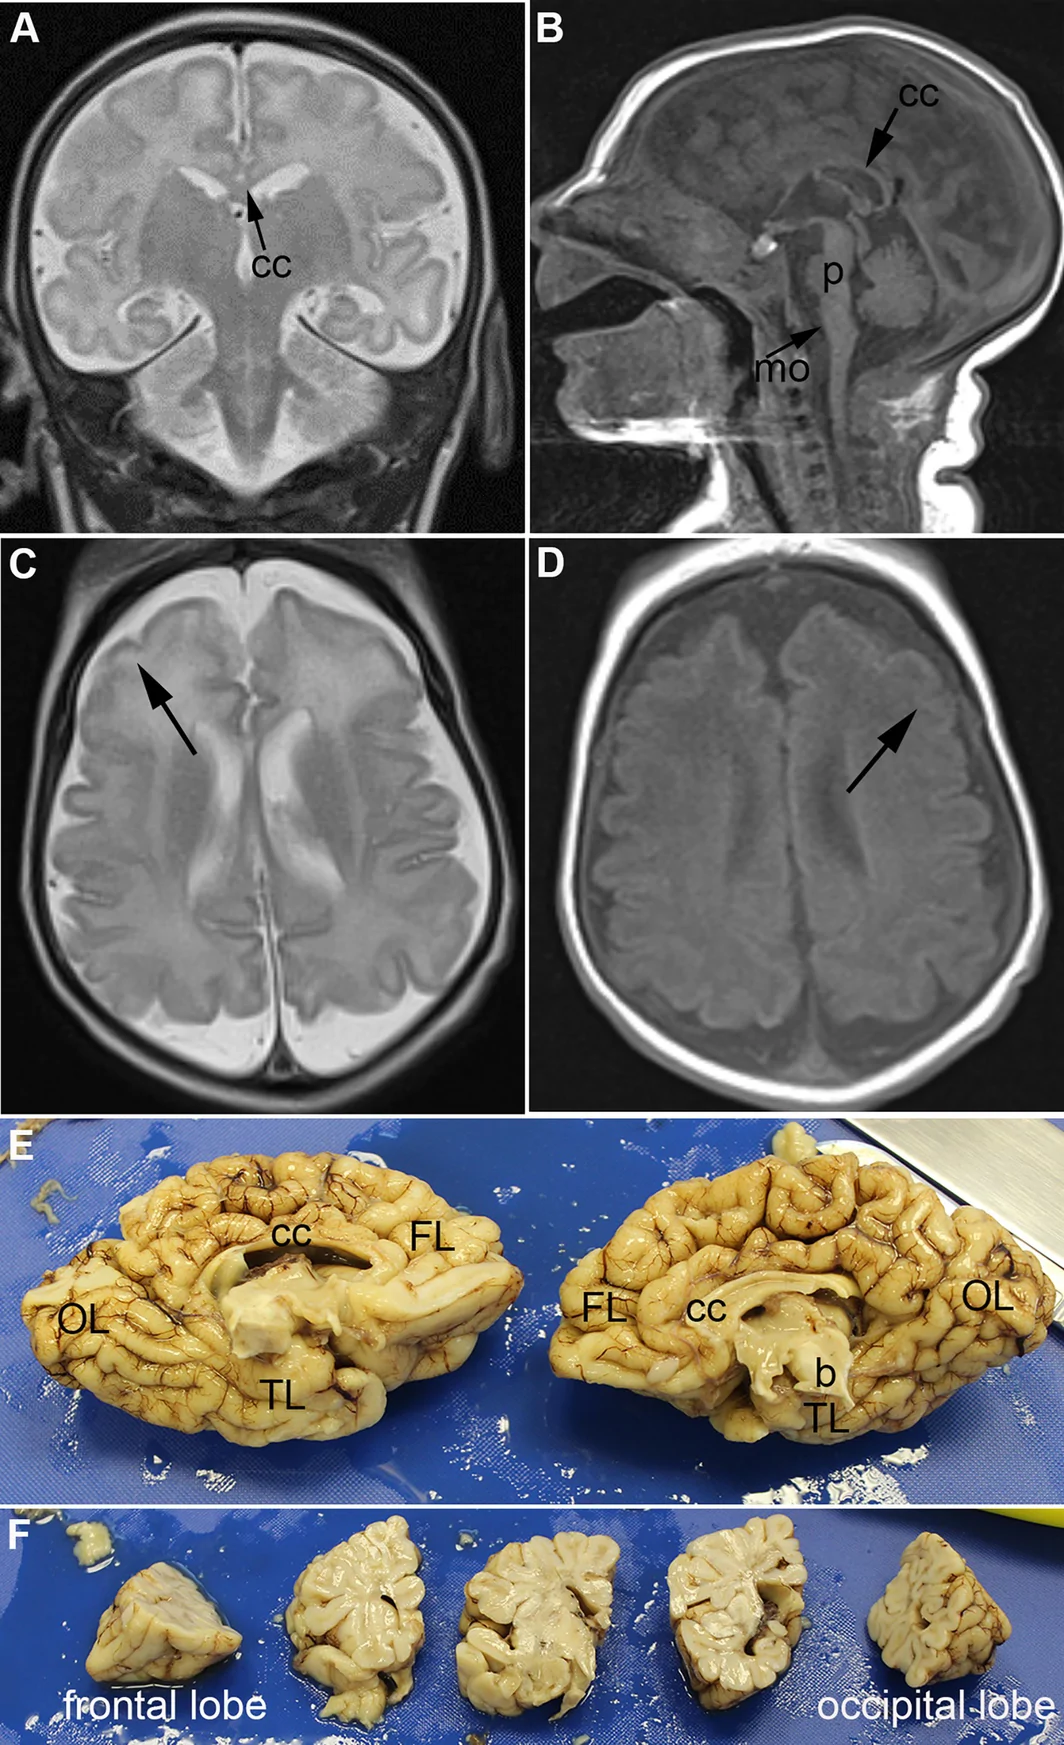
MRI in the second week of life and postmortem macroscopic photographs (6 months of age). MRI taken in the second week of life showed a simplified tertiary gyrification pattern (A, arrows in C and D), a thinner corpus callosum (A, B, also visible in E) than expected, and hypoplastic medulla oblongata and pons (B). The ventricular system was normally positioned and of normal size, without evidence of hydrocephalus (A–D). The medial view of the telencephalic hemispheres is shown in (E), while coronal slices are displayed in (F). MRI sequences: (A) T2 coronal plane, (B) T1 mid‐sagittal plane, (C) T2 axial plane, (D) T1 axial plane. b, brainstem; CC, corpus callosum; FL, frontal lobe; mo, medulla oblongata; OL, occipital lobe; p, pons; TL, temporal lobe.
